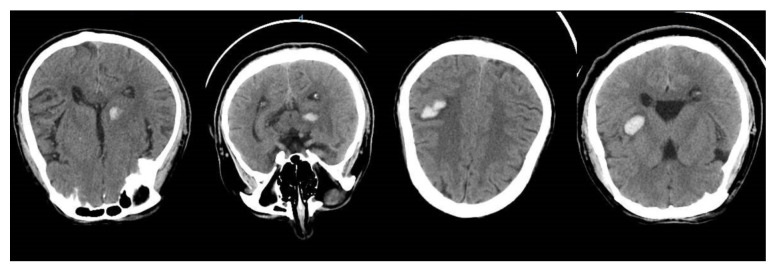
Example ICH CT images from the Guilin Hospital dataset.
